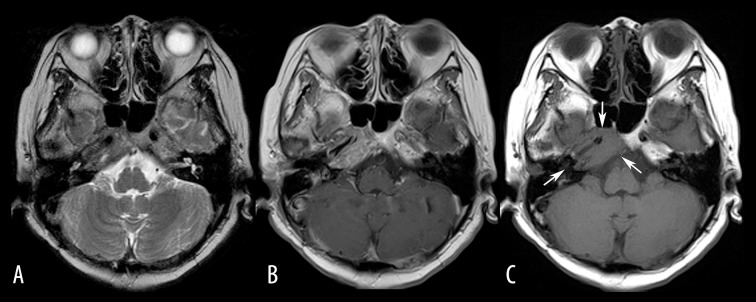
Bone metastasis to the skull base in a 53-year-old woman with a history of breast cancer. (A, B) On axial T2-weighted (A) and contrast-enhanced T1-weighted (B) MR images, MR signals from the metastatic bone tumor of the right petrous apex resemble those from the bone marrow in the contralateral normal petrous apex. (C) Non-contrast, T1-weighted (C) MR image most clearly delineates the hypointense tumor (arrows).
